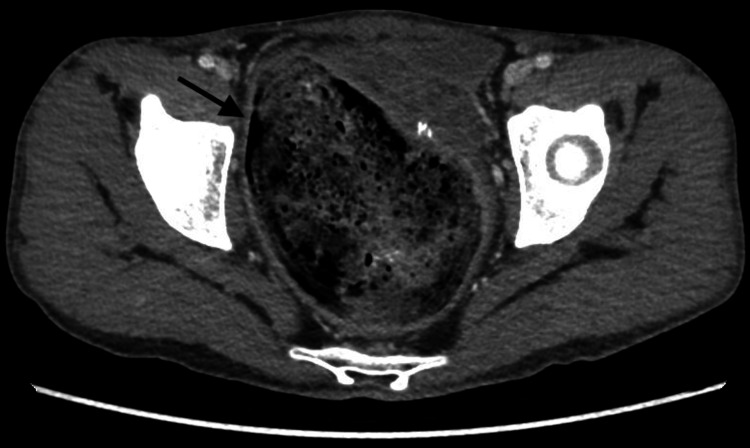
Axial CT scan with contrast showing significant focal dilatation of the rectum with a large fecaloma inside, measuring about 12.2 cm in length, 8 cm in width, and 10 cm in height. Image of a huge dilated rectum loaded with feces.
